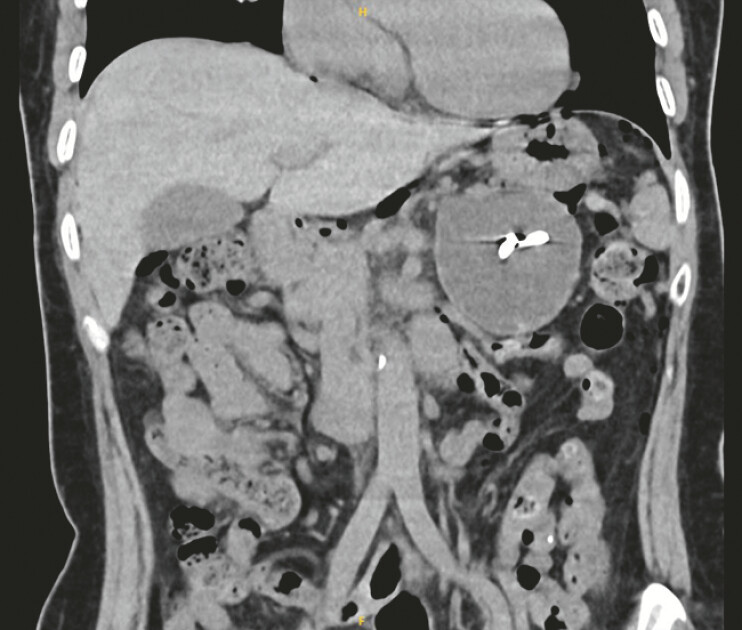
Abdominal computed tomography scan view showing complete migration of the first stent into the pseudocyst cavity.
